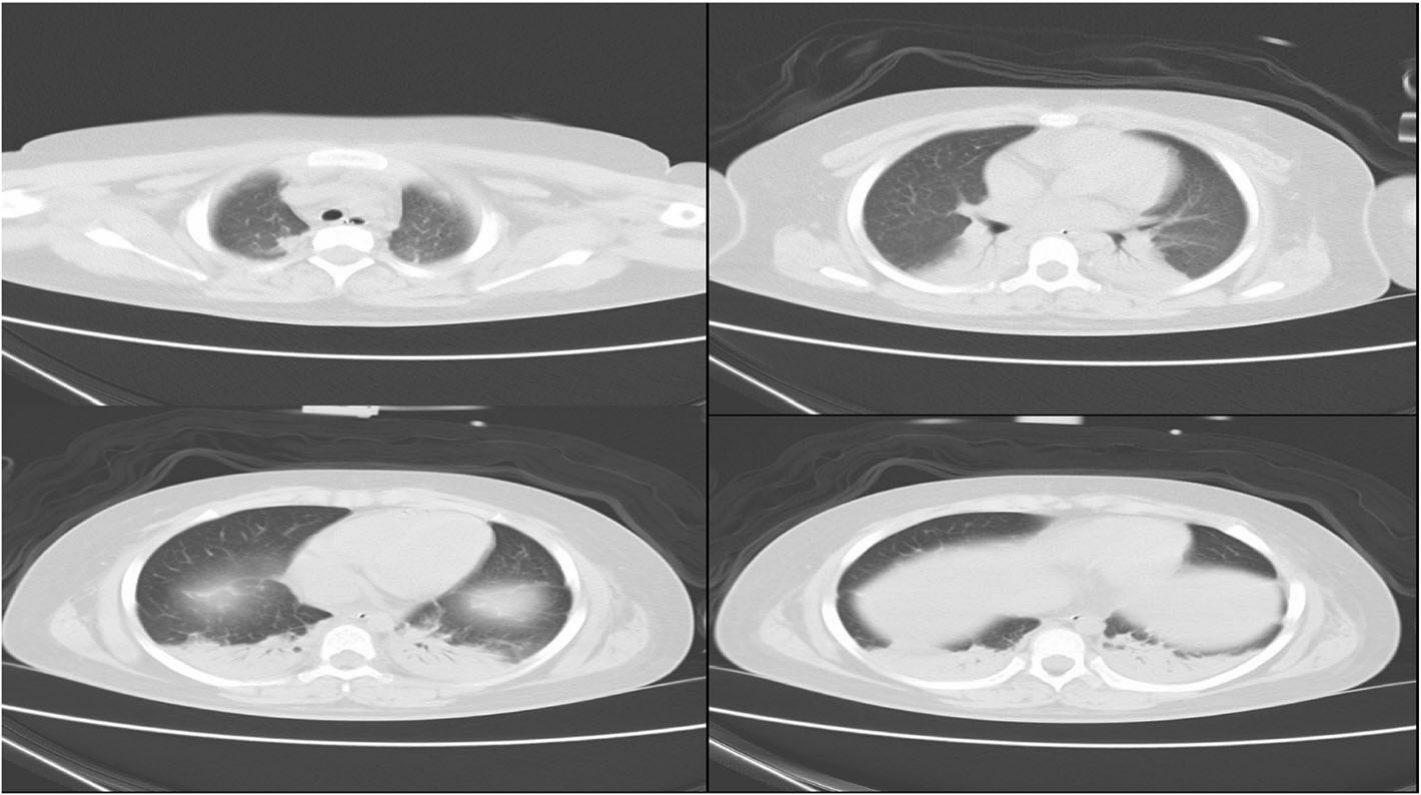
Chest CT of 6th April (after 48 h of ICU supportive care). This revealed the most of lung field was normal except the bilateral lower lobe alveolar infiltrates and consolidation
